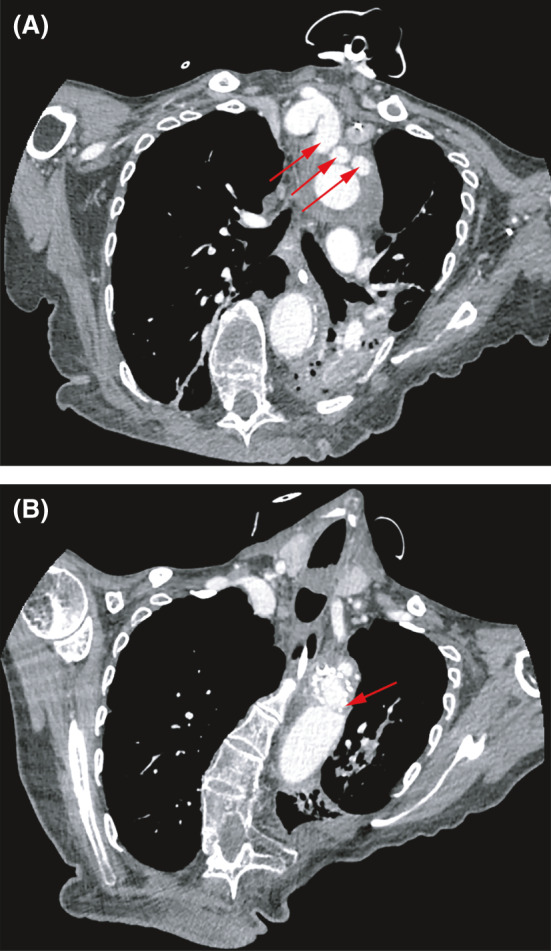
Chest CTA with contrast showing postoperative total aortic arch replacement with Cor‐Knot automated fastening. A) Trifurcated graft anatomy displayed. B) Distal aortic anastomosis with Cor‐Knot automated fastening
